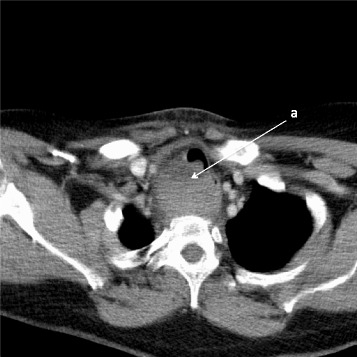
CT Neck showing large mass with a hypodense fluid level (a)
